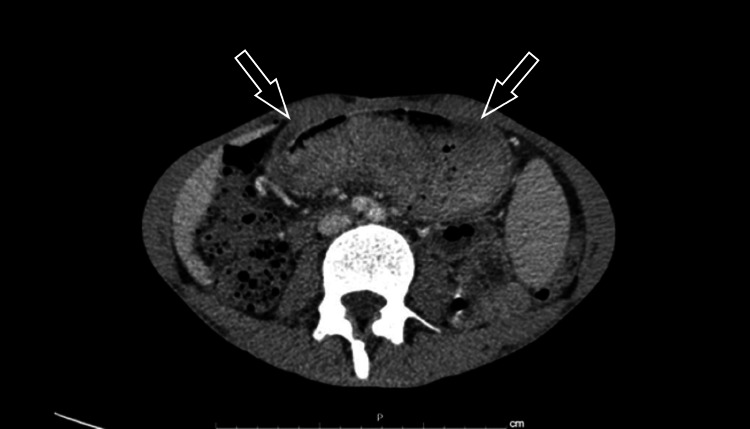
CT reveals a large exophytic mass in the gastric body and antrum with infiltration of the adjacent fat.
